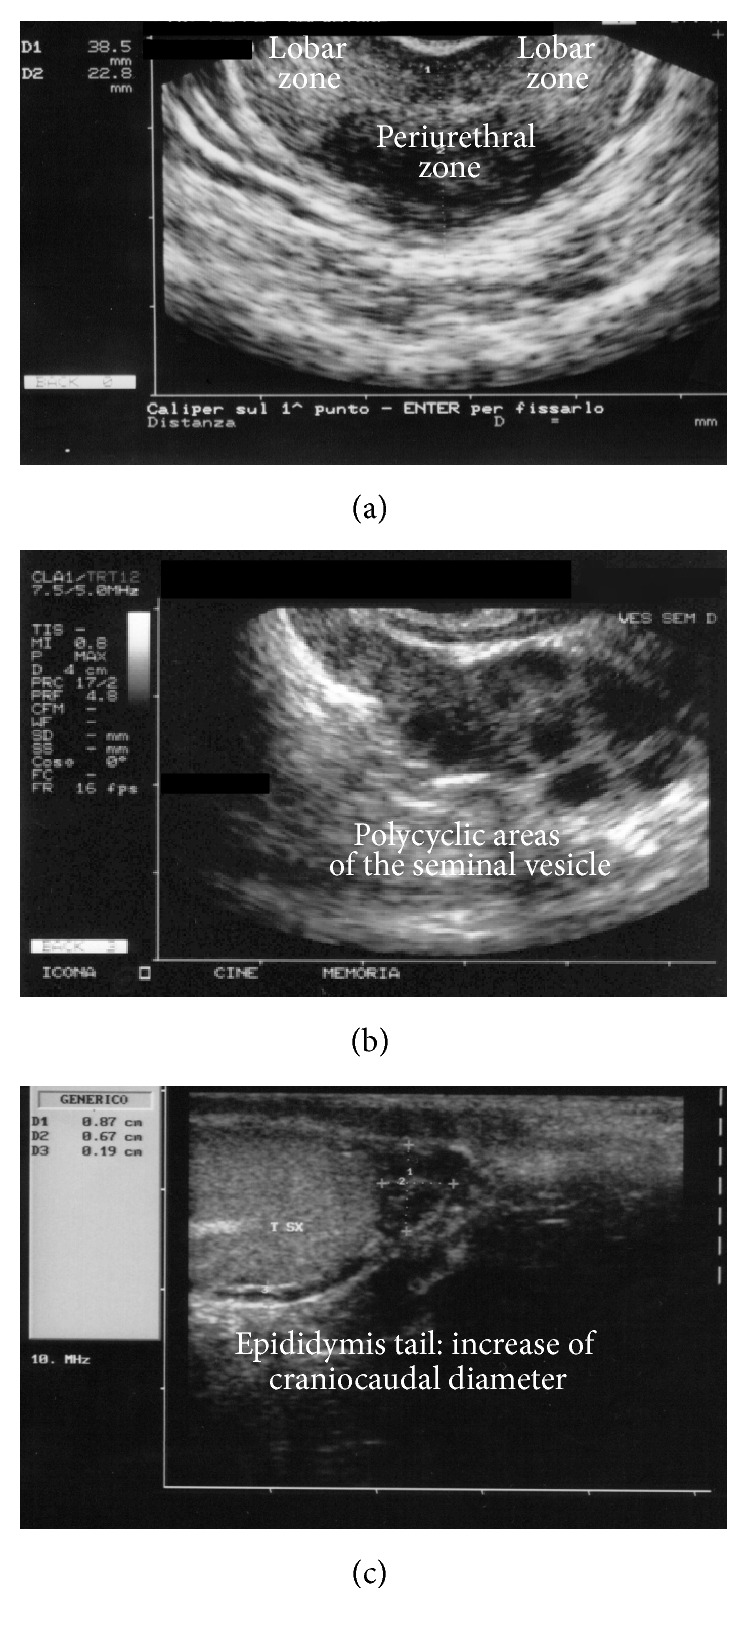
Examples of ultrasonographic suggestive images in patients with MAGI. (a) US subdivision of the prostate in periurethral and lobar regions. (b) Chronic vesiculitis. (c) Chronic epididymitis.
